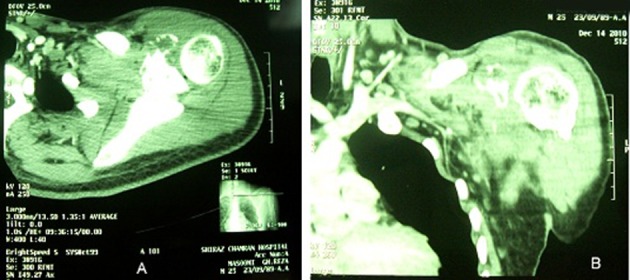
(A) CT scan of the scapula, (B) MRI of the scapula
